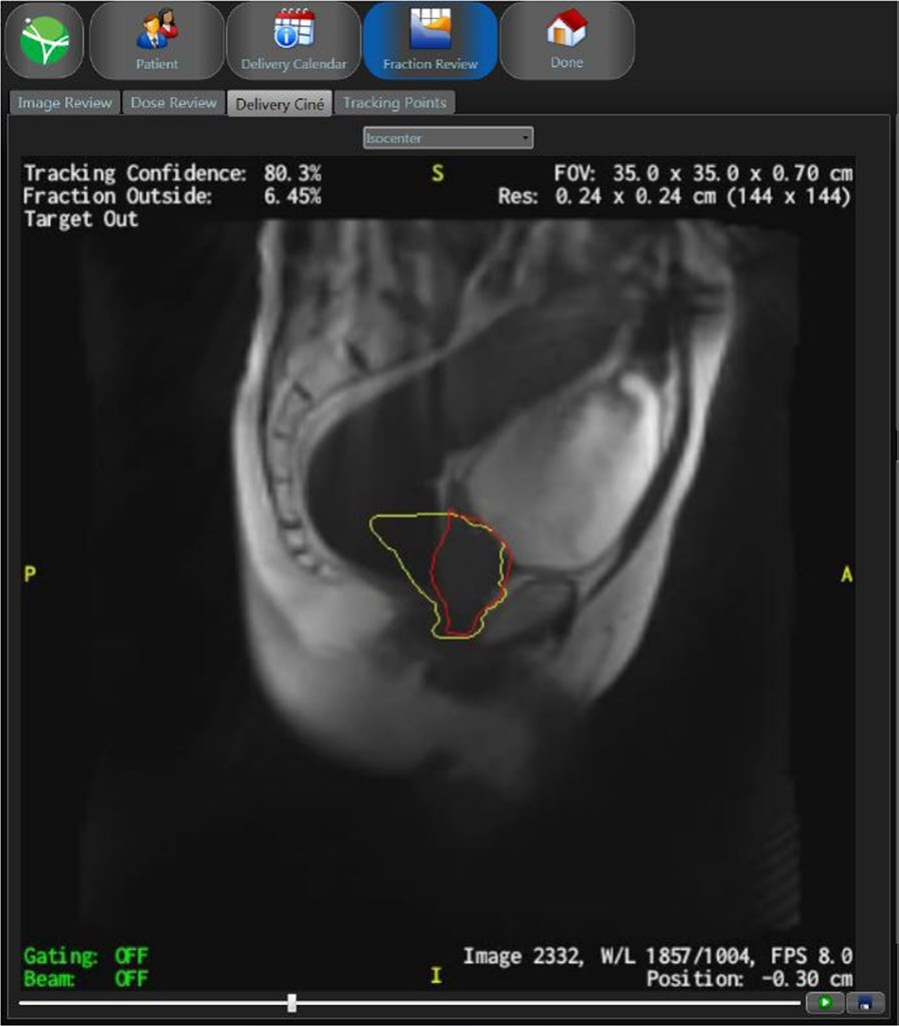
Cine MRI sequence. Yellow structure: Gating margin (CTV + 3 mm). Red structure: deformably registered gating target. This screenshot is showing the instance when a large amount of rectal air is moving the prostate out of the gating margin, automatically shutting off beam delivery
